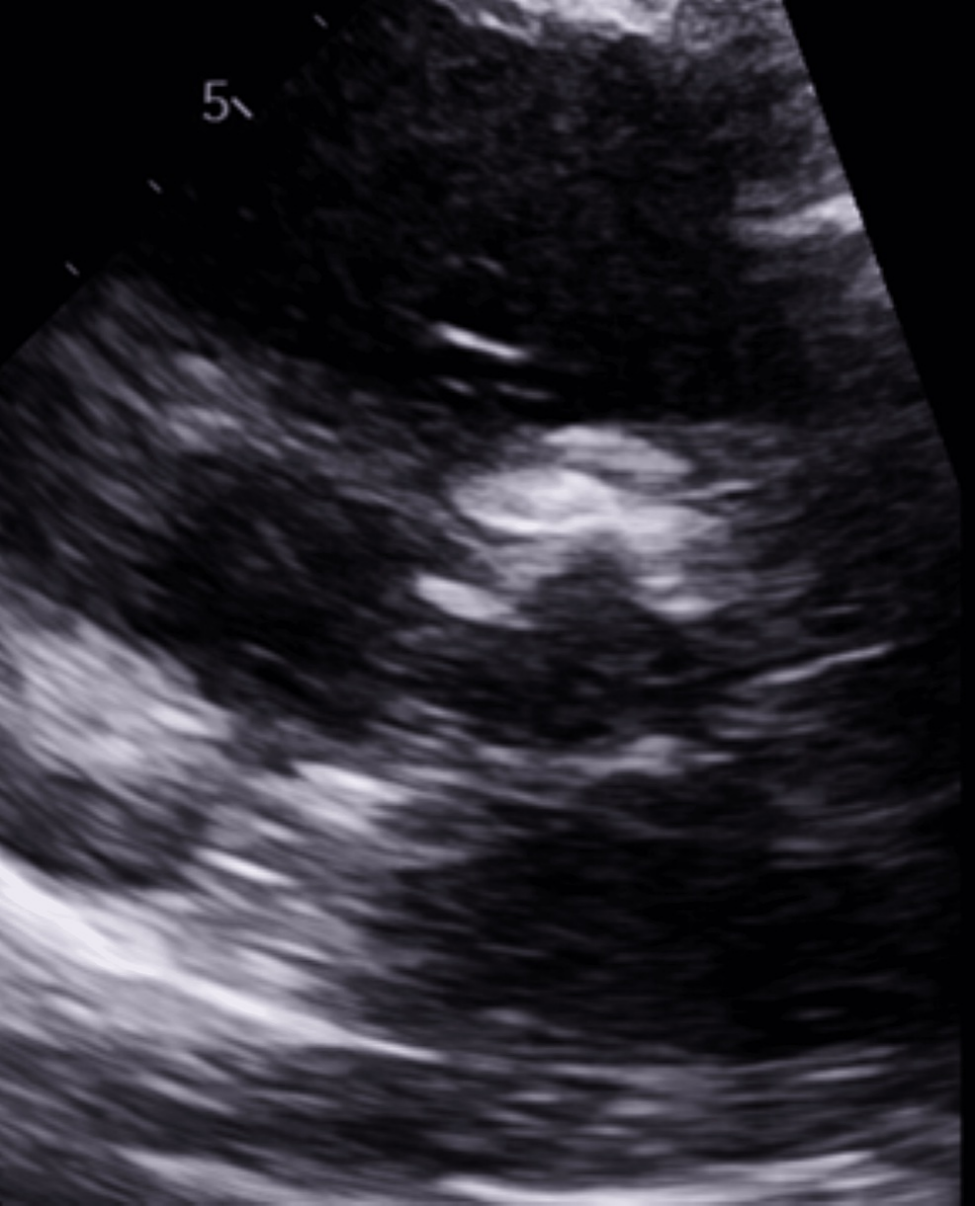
Post-TAVR Parasternal Short-Axis View Image taken one day following procedure TAVR: transcatheter aortic valve replacement
